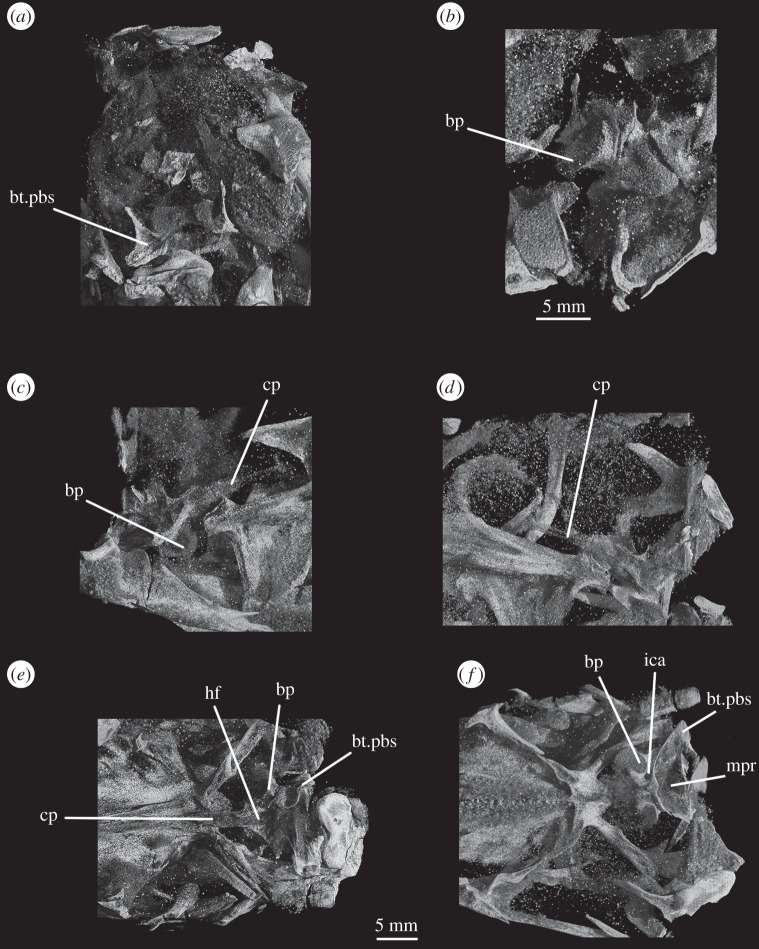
CT reconstructions of braincase of SAM-PK-6047A in (*a*) posterior, (*b*) anterior, (*c*) right lateral, (*d*) left lateral, (*e*) dorsal and (*f*) ventral views. For abbreviations, see table 1.
